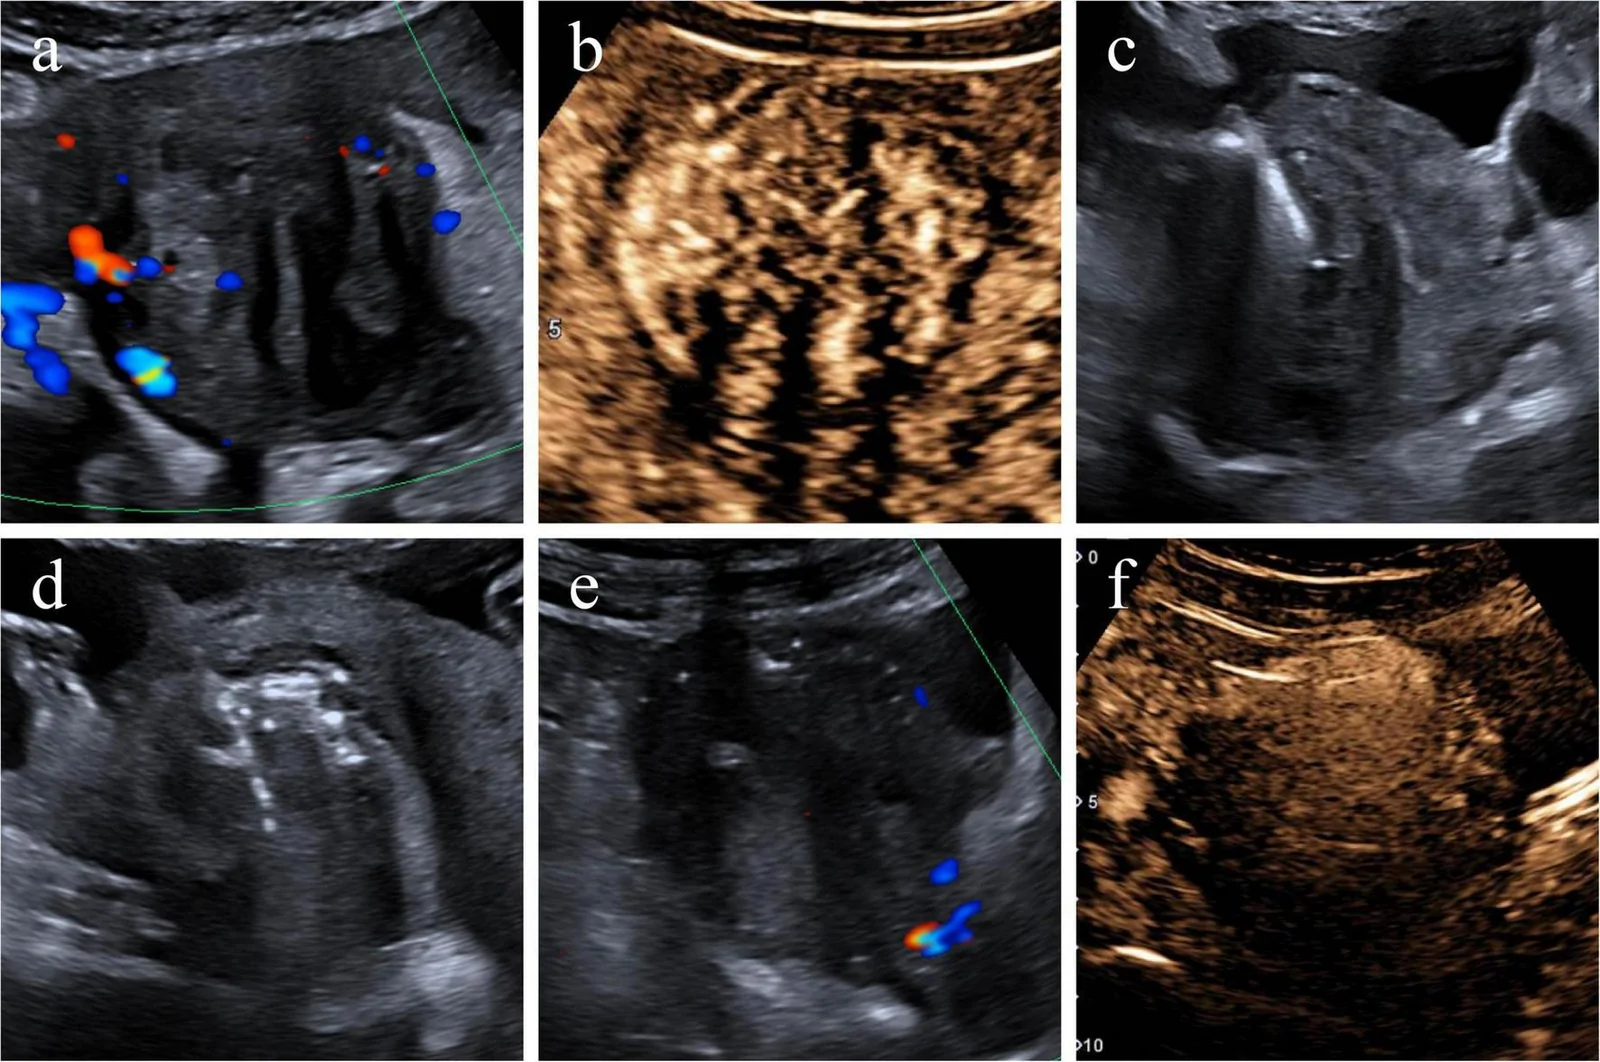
Intraoperative sonographic image during MWA. (a) Pre-ablation color Doppler ultrasound demonstrated blood flow signals within and around the fibroid. (b) Pre-ablation CEUS revealed pronounced enhancement of the fibroid. (c) Pre-ablation needle biopsy. (d) Intraoperative ablation zone exhibited hyperechoic changes. (e) Post-ablation color Doppler ultrasound showed near-complete disappearance of blood flow signals. (f) Post-ablation CEUS exhibited no significant enhancement within the ablation zone.
